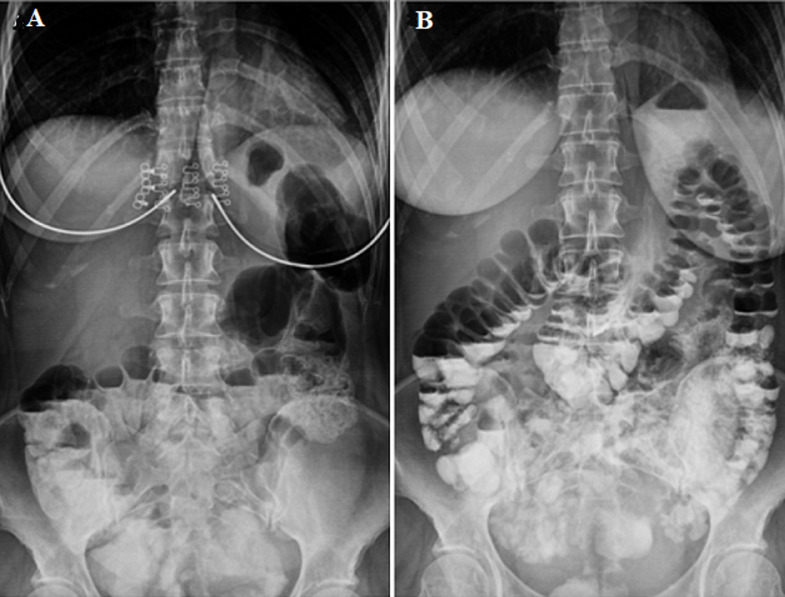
abdominal X-ray of the patient: A) on admission to the emergency department; B) after oral administration of gastrografin gastroenteral solution
